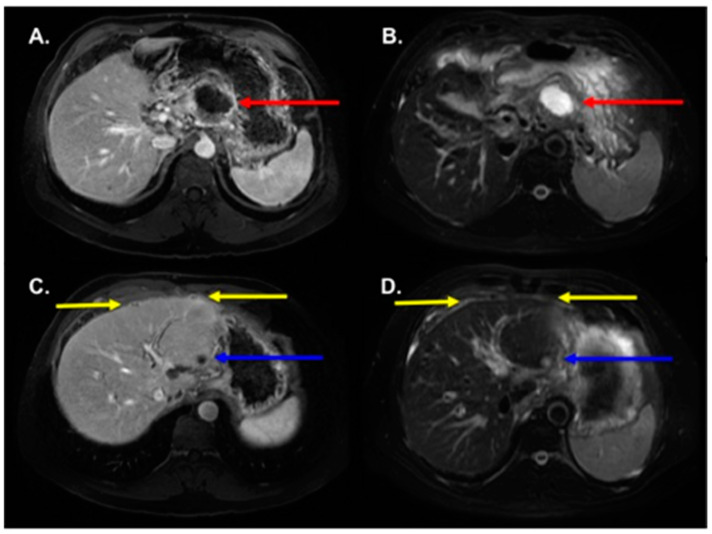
A 48-year-old male with a necrotic mass in the pancreatic body. (A) Axial T1 fat-suppressed portal venous phase image demonstrates central hypo-enhancement (red arrow). (B) Axial T2 fat-suppressed image shows associated hyperintensity (red arrow). (C,D) Hypo-enhancing, T2 hyperintense lesions are seen within the liver (blue arrows) and along the anterior hepatic capsule (yellow arrows). Endoscopic ultrasound biopsy revealed primary PDAC with necrosis.
